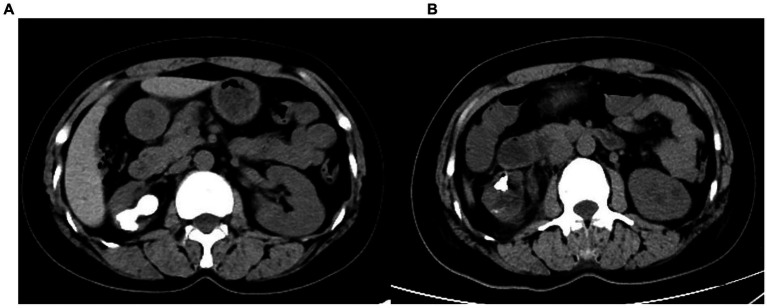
CT of (A) before and (B) after surgery.
